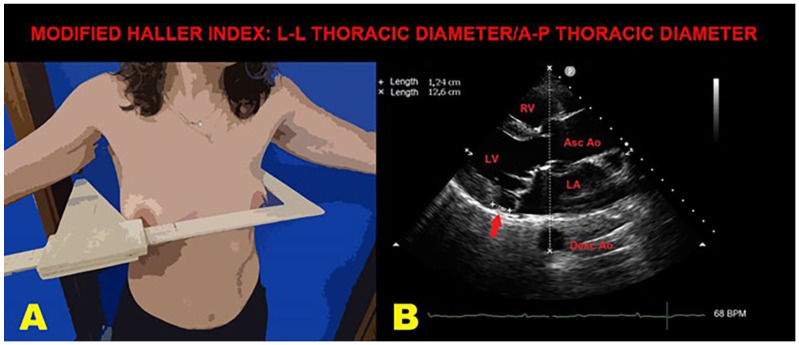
An example of MHI assessment in an MVP individual with MAD. (A): The L-L thoracic diameter, measured by using a rigid ruler in centimeters coupled to a level, placed at the distal third of the sternum, at the point of maximum depression of the sternum. (B): The A-P thoracic diameter (white dotted line), obtained from the echocardiographic parasternal long-axis view by measuring the distance between the true apex of the sector and the posterior wall of the descending thoracic aorta, visualized behind the left atrium. MAD is indicated by the red arrow. Ao, aorta; A-P, antero-posterior; Asc, ascending; Desc, descending; LA; left atrium; L-L, latero-lateral; LV, left ventricle; MAD, mitral annular disjunction; MHI, modified Haller index; MVP, mitral valve prolapse; RV, right ventricle.
